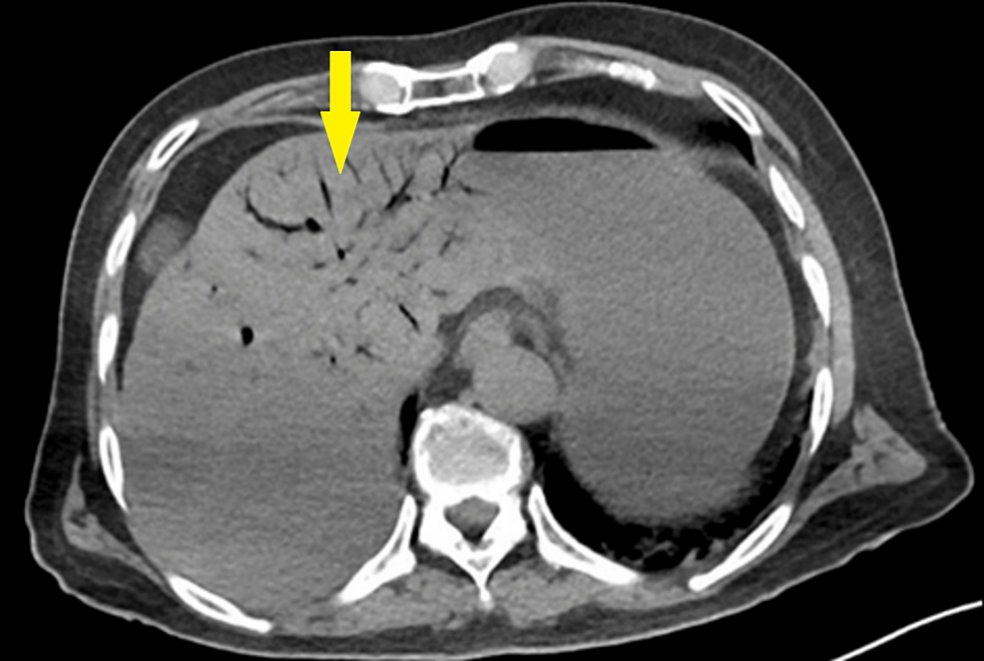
CT scan of the abdomen revealing the presence of air within the portal vein, predominantly observed on the right side of the liver.
